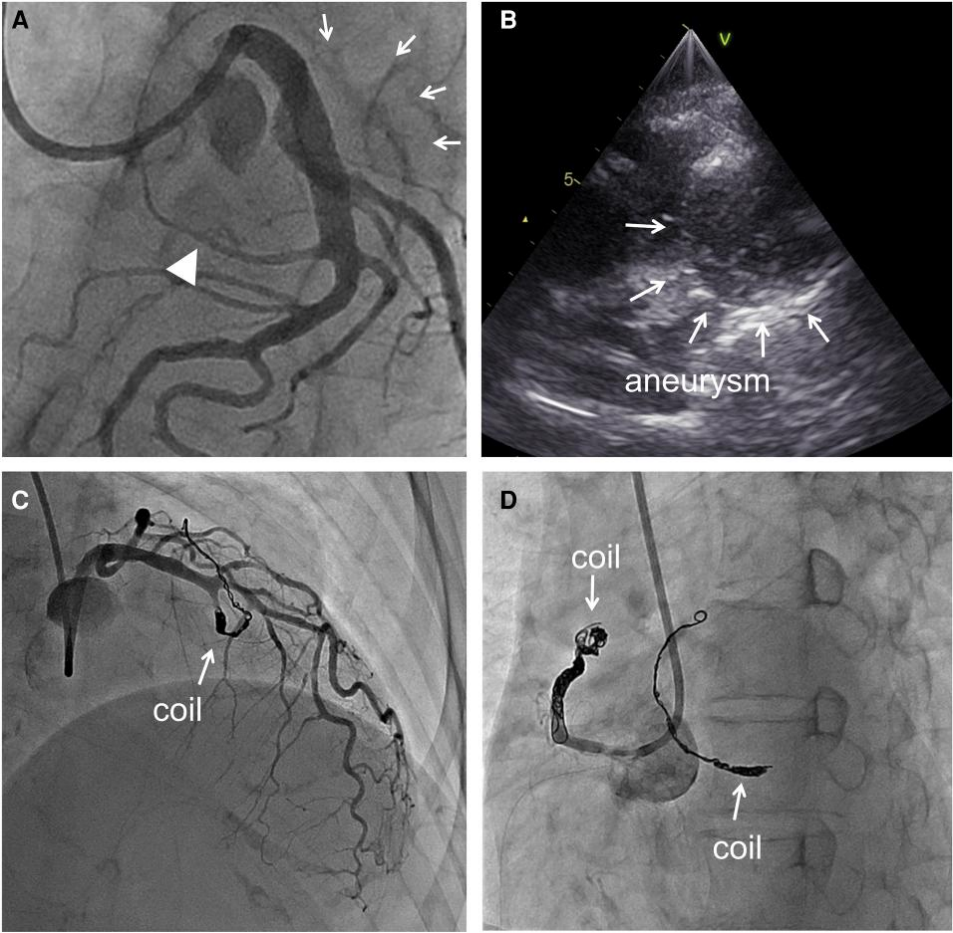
(*A*) Coronary angiography on the day of embolization. The feeding artery via the left descending artery (LAD) was occluded midway (triangle), and the aneurysm showed minimal contrast agent (*B*) Echocardiography on the catheter bed. Isoechoic-to-hyperechoic solid echo inside the aneurysm, and a blood flow signal was absent. (*C*) After embolization of the feeding artery via the LAD. (*D*) After embolization of the coronary-to-pulmonary artery fistula of the conus branch.
